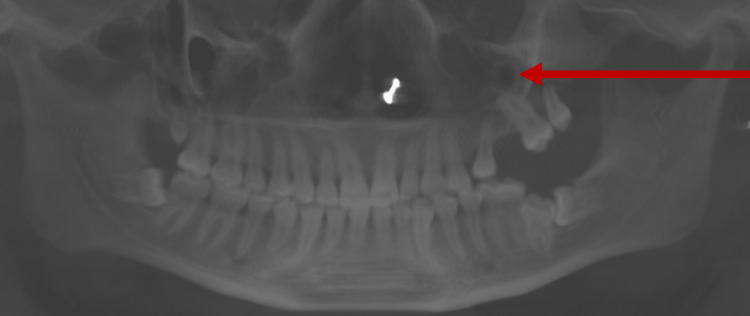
Arrow in panoramic reconstruction generated from cone-beam computed tomography showing tumor displacing the teeth.
